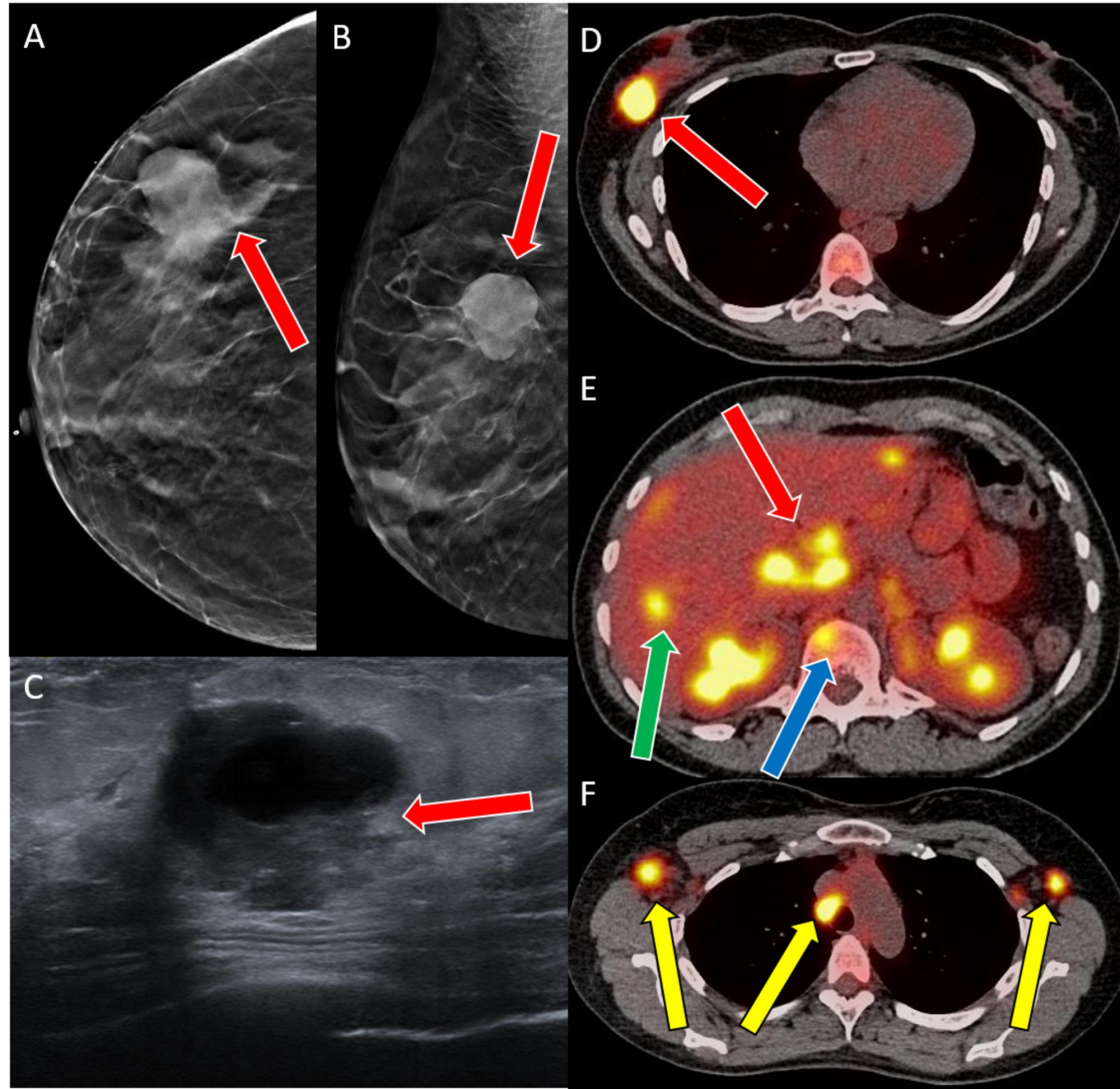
Case 1 imaging findings A, B: Craniocaudal and mediolateral oblique tomosynthesis views of the right breast show an oval high-density mass (red arrows) with partially spiculated margins. C: Transverse ultrasound shows an oval mixed solid and cystic mass (red arrow) with partially indistinct margins. D-F: PET demonstrates (D) a hypermetabolic right breast mass (red arrow), (E) porta-hepatis lymphadenopathy (red arrow), hepatic (green arrow) and bony metastasis (blue arrow), (F) mediastinal and bilateral axillary lymphadenopathy (yellow arrows). PET: positron emission tomography
